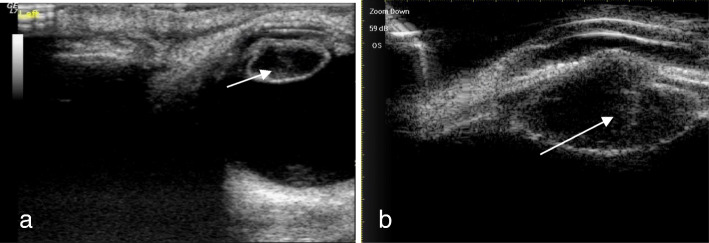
a The false negative image taken with 14-MHz + THI. The anterior capsule of the lens is ruptured, the echo of the PC in the lens is regular and continuous, passage is seen in the lens (short arrow), the PC is not penetrated, and the PC is mistaken for being intact. b: The false negative LFUBM image. The ultrasound performance is similar to that shown in a (long arrow)
